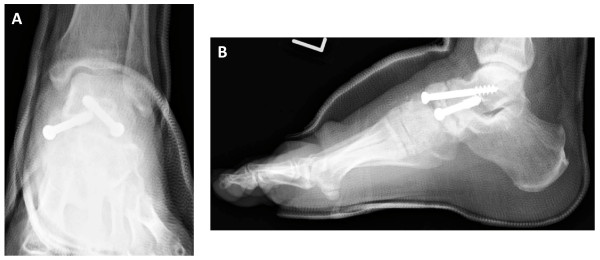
Postoperative radiographs showed fixation with two screws. (A) Anteroposterior radiograph. (B) Lateral radiograph.
